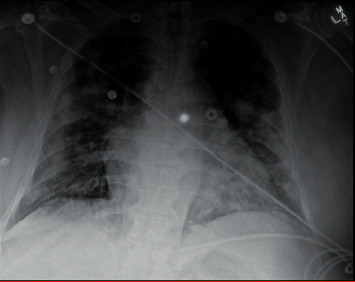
Chest X-ray on day 14 showing worsening bilateral infiltrates.
